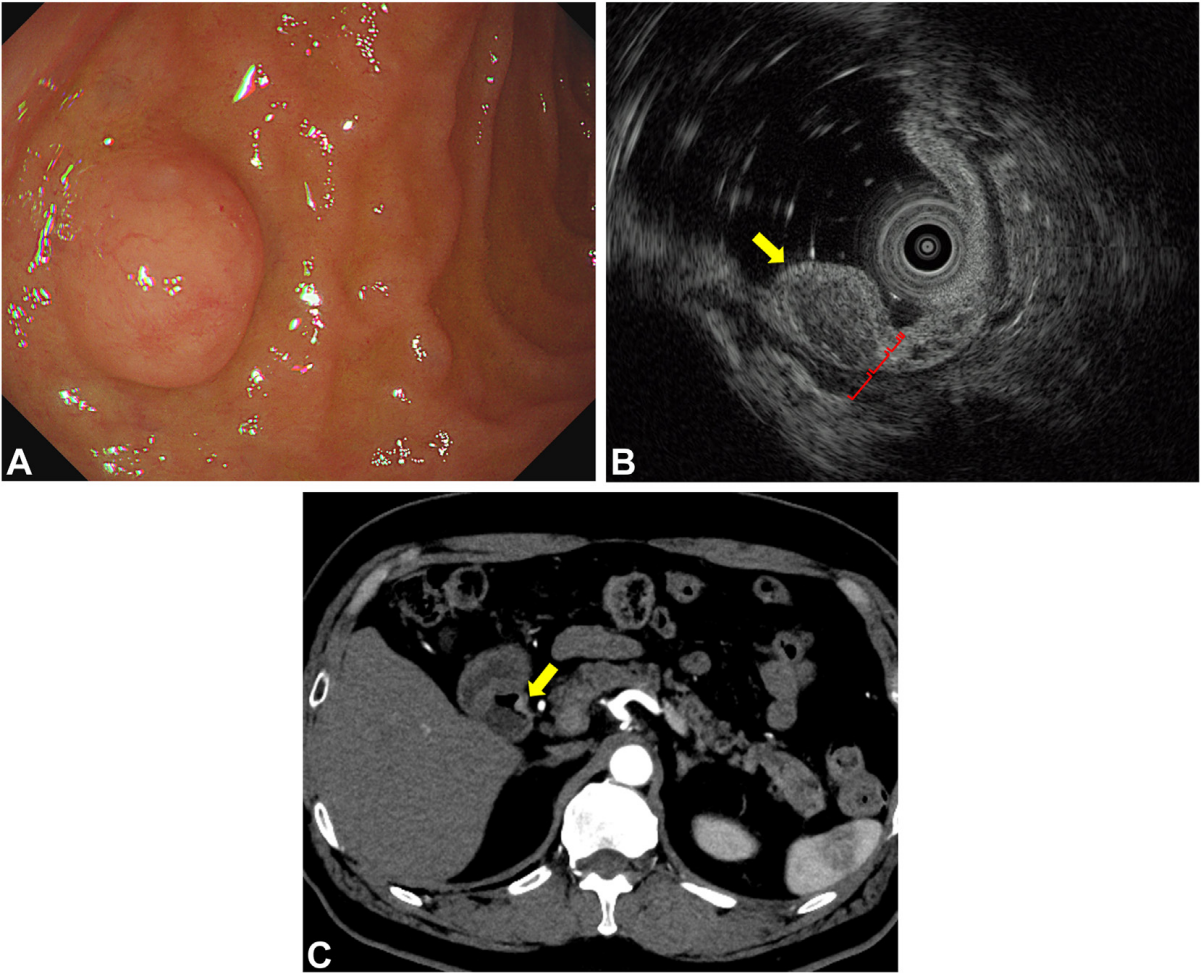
Preoperative diagnostic images. A, Esophagogastroduodenoscopy showing an 8-mm semicircular tumor covered with normal mucosa in the anterior wall of the duodenal bulb. B, Endoscopic ultrasonography identifying heterogeneous hypoechoic mass (*yellow arrow*) in the submucosal layer (third layer) without invasion into the muscular propria (fourth layer). Each layer of the duodenal wall is indicated with a square bracket. C, Enhanced computed tomography demonstrating a 6-mm enhanced tumor (*yellow arrow*) in the duodenal bulb without any lymphadenopathies.
